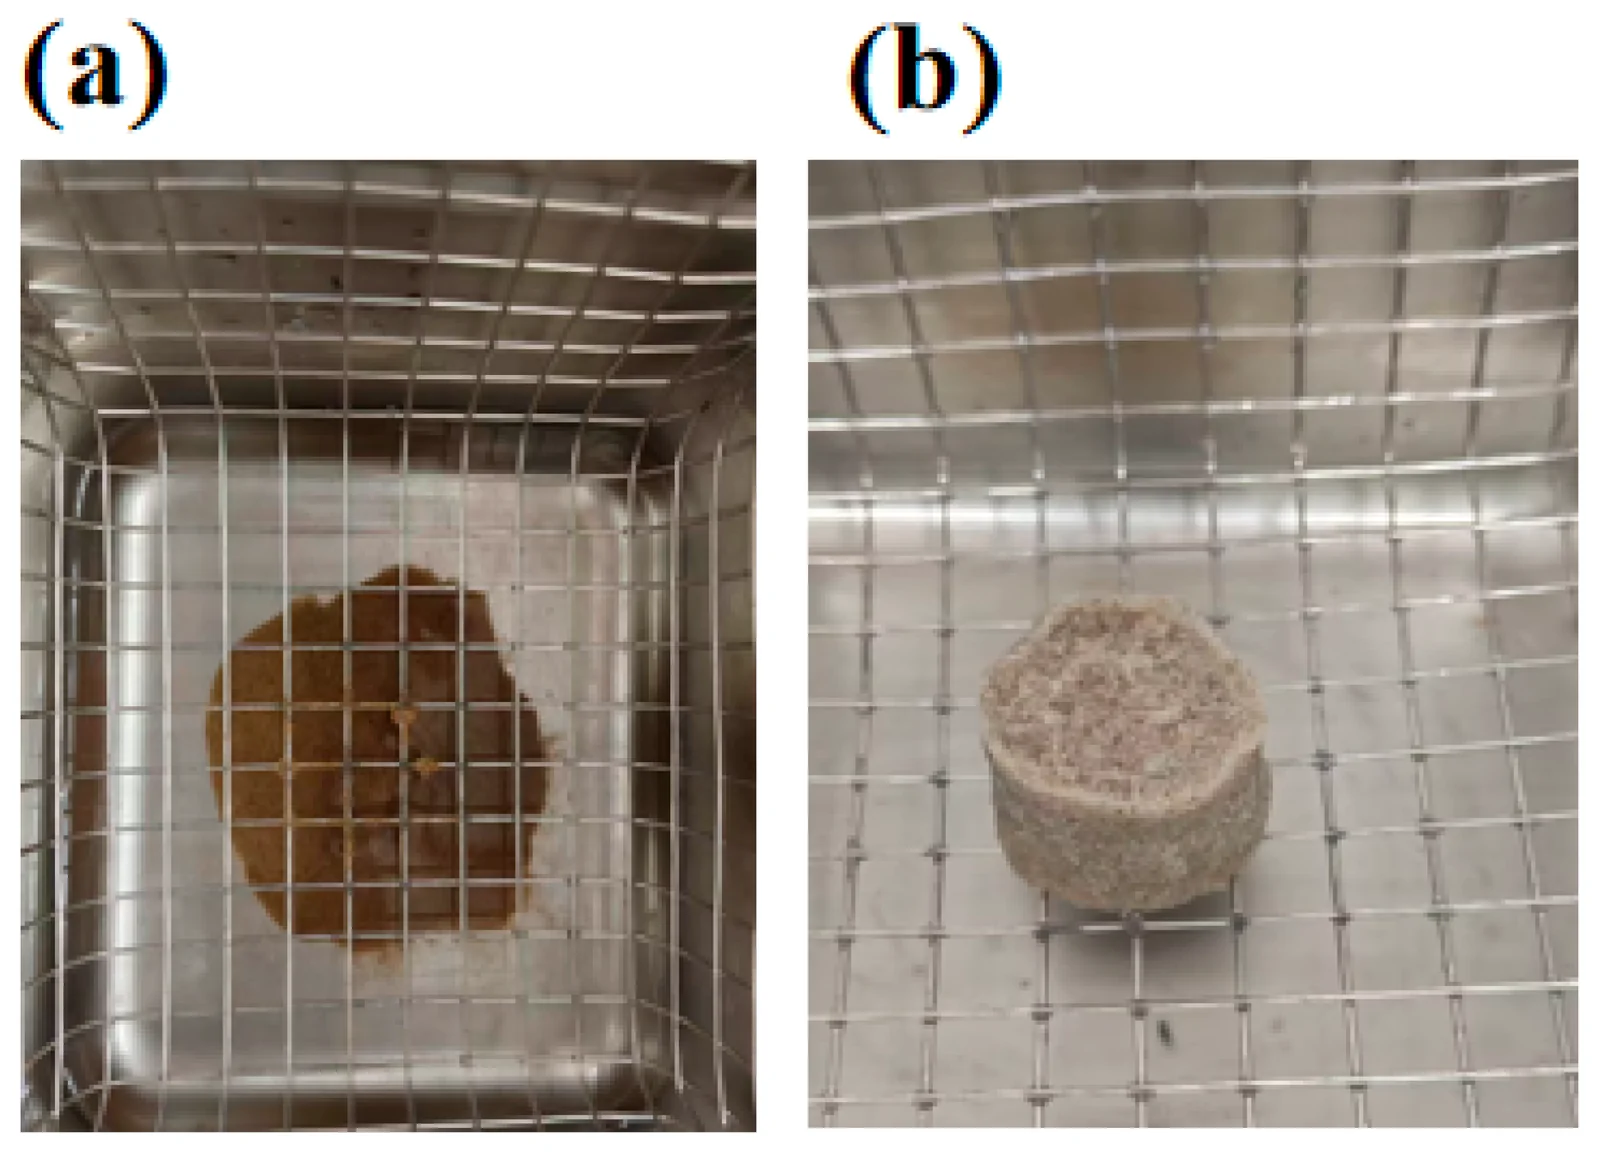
Morphological of RQS (a) and MRQS (b) aggregates after ultrasonic collapse test.
